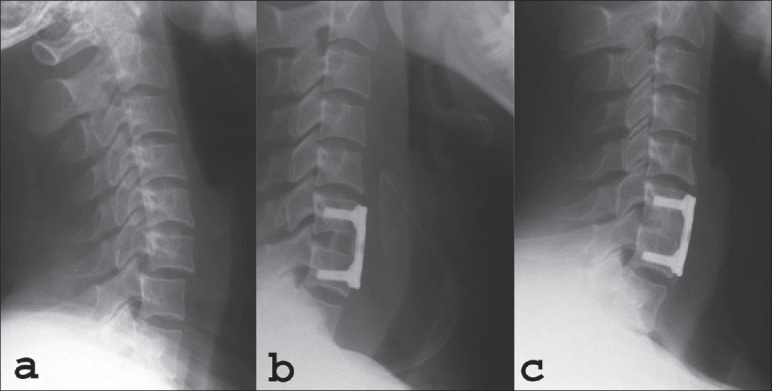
Lateral x-ray (a) cervical spine shows unifacetal dislocation C6/C7. Postoperative lateral x-ray (b) cervical spine of the same patient shows graft and plate in situ. Lateral x-ray (c) cervical spine of the same patient shows graft incorporation with subsidence at six months
